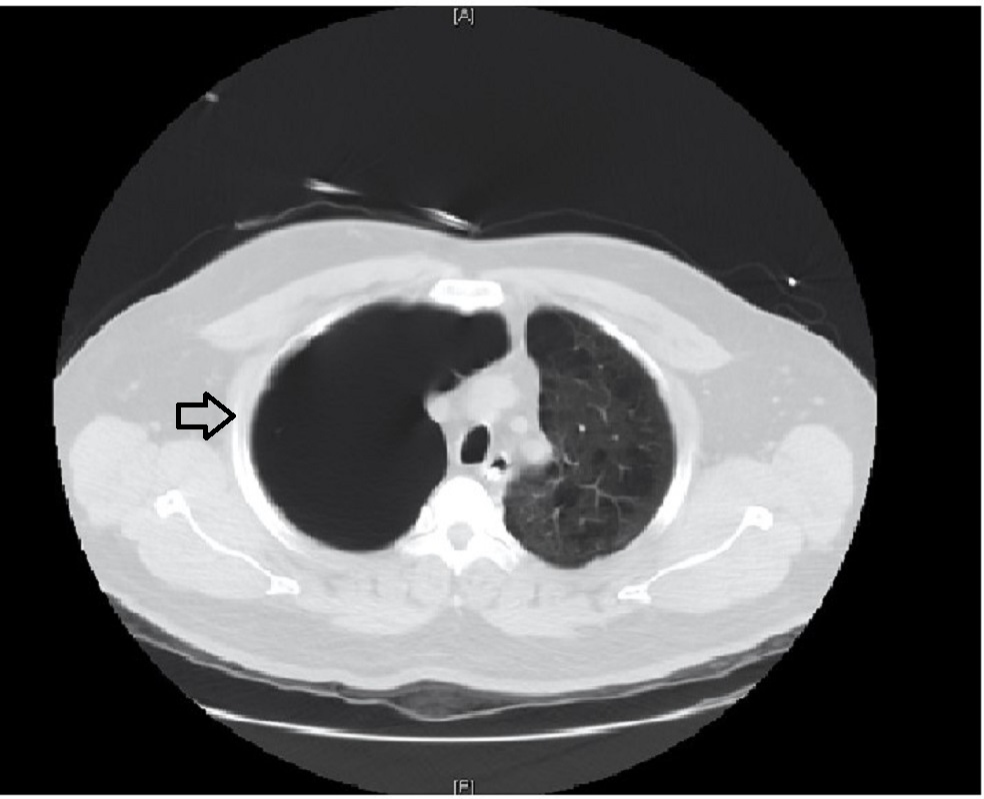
Axial computed tomography (CT) of the chest on admission. A large bulla (black arrow) occupying the right hemithorax.
